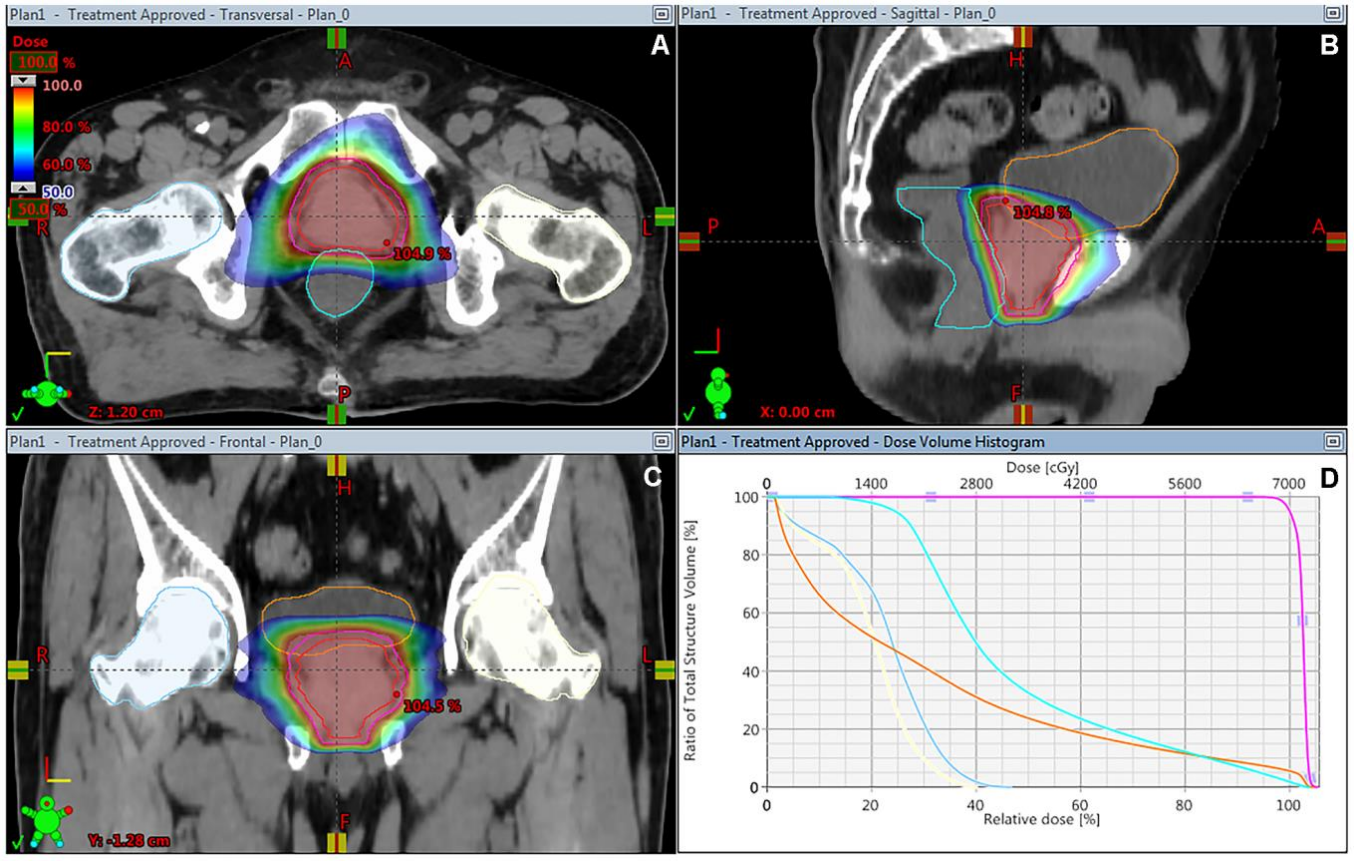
Dose distribution on the transverse (A), sagittal (B) and coronal (C) CT imaging, and DVH (D) of treatment planning of hypofractionated VMAT for patients with prostate cancer. DVH, dose–volume histogram; VMAT, volumetric modulated arc radiotherapy.
